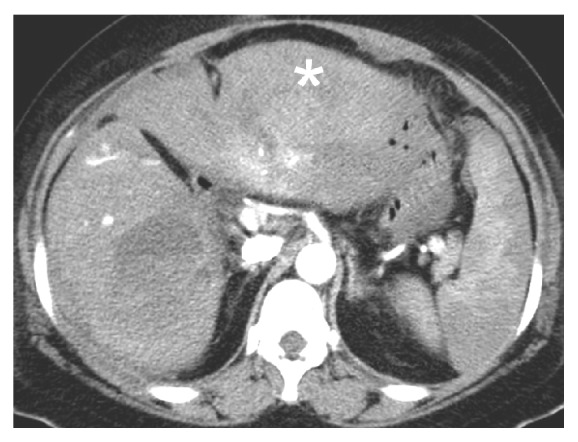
The computed tomographic angiography of abdomen 3 months later showed complete resolution of subscapular hematoma (asterisk). A residual hematoma over right lobe of liver was noted but without any clinical manifestation.
